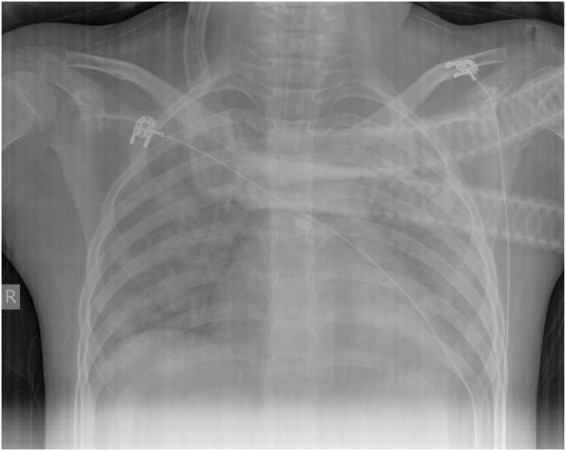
X-ray of the patient. Diffuse exudative lesion in both lungs (day of admission).
